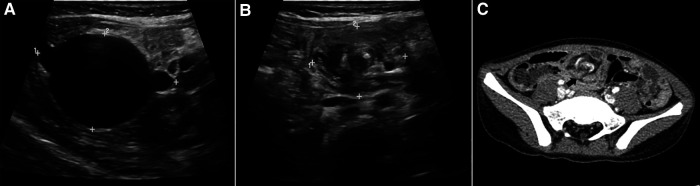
Imaging of MCs complicated with volvulus. (A) Sonographic imaging demonstrating a well-marginated cyst; (B) proximal mesentery with the alternating rings (asterisk) of low and high echogenicity due to volvulus (concentric circle sign); (C) enhanced CT scan revealing the whirlpool sign (asterisk) of the mesenteric artery and vein (arrow).
